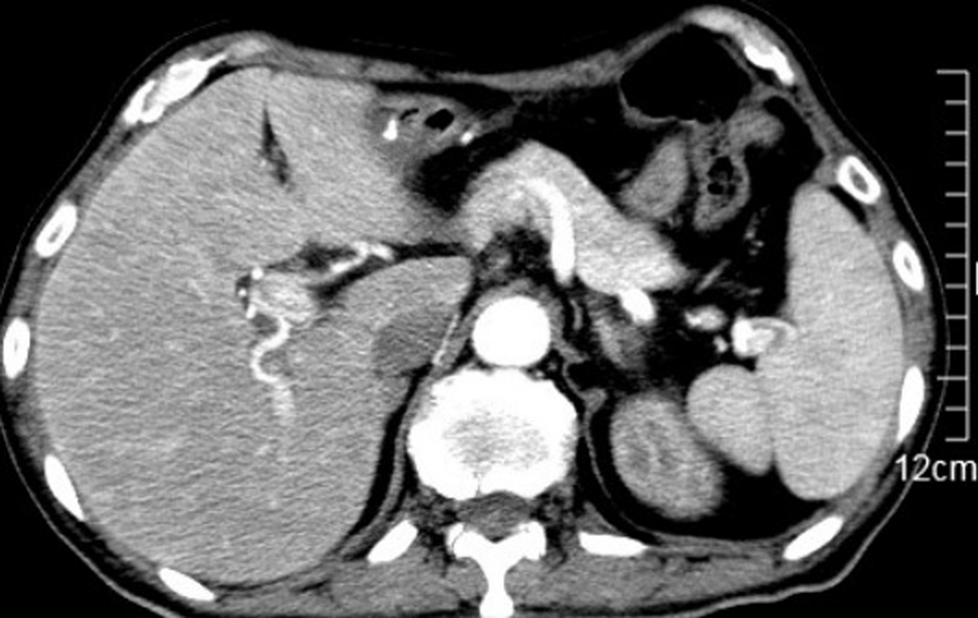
Contrast-enhanced abdominal computed tomography showing heterogeneous enhancement of liver density.
